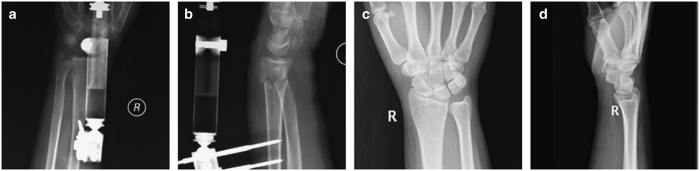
Radiographs of the distal radius with a comminuted intra-articular fracture after external fixation. (a and b) Anteroposterior and lateral radiographs after one month, (c and d) Anteroposterior and lateral radiographs after one year.
